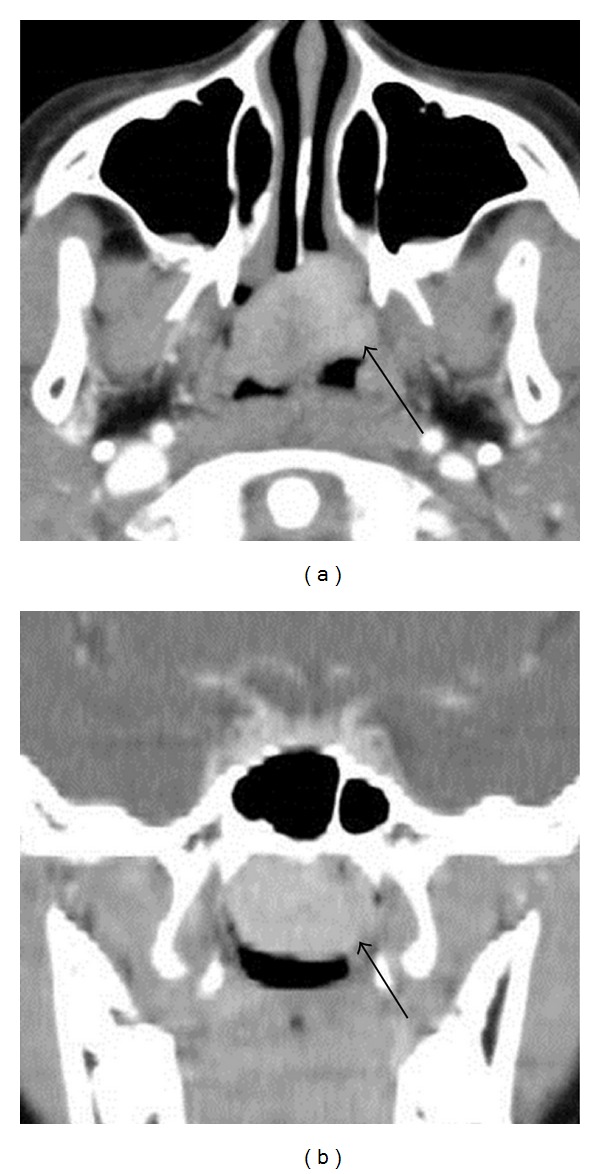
Axial (a) and coronal (b) computed tomography scans with contrast medium showing a soft tissue mass in the nasopharynx with homogeneous enhancement (arrows).
